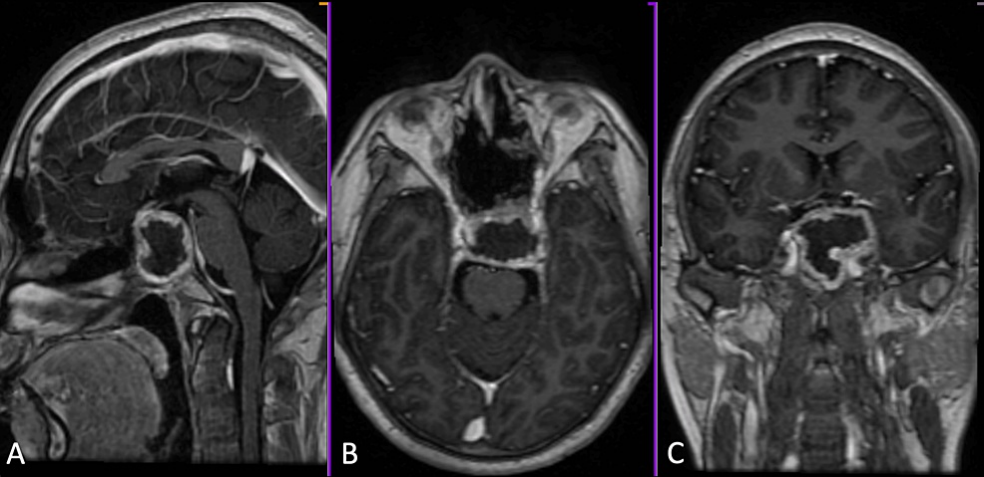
Post-radiation therapy, brain MRI T1 with contrast showed an interval decrease in the size of the mass, measuring 2.7 x 3.8 x 3.7 cm (AP x W x CC), which now demonstrates intralesional necrosis. (A) Sagittal view, (B) Axial view, and (C) Coronal view.
